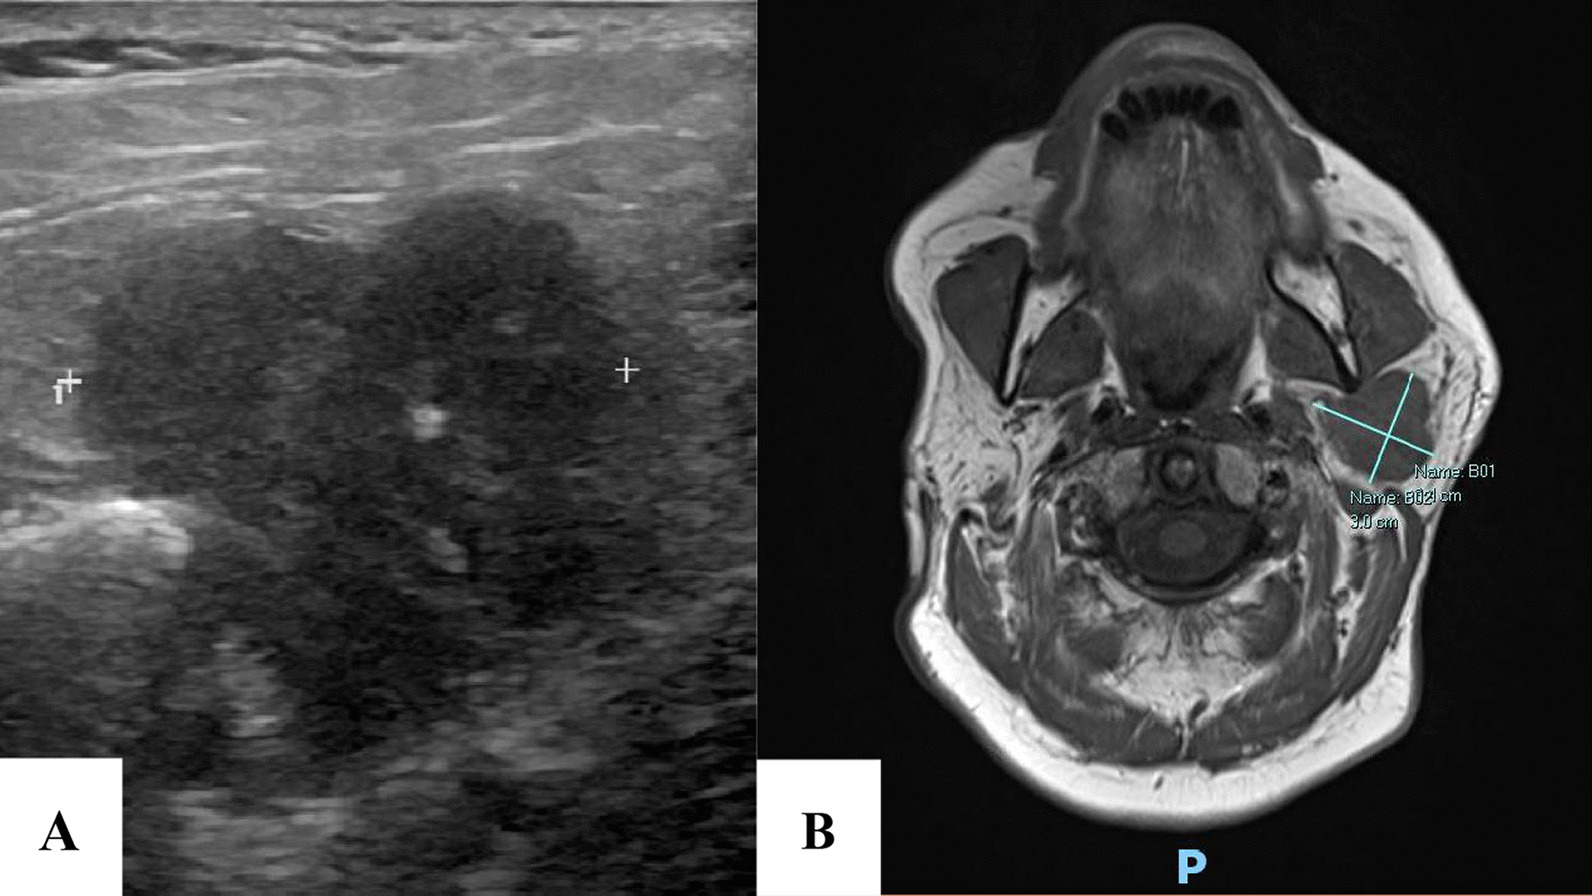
Ultrasonography (A) showed a hypoechoic mass in the left parotid, 2.8 cm in greatest dimension with poorly defined borders. Magnetic Resonance Imaging (B) showed a well-defined cystic and/or necrotic mass involving the left parotid gland, 3.4 × 3.0 × 3.0 cm
